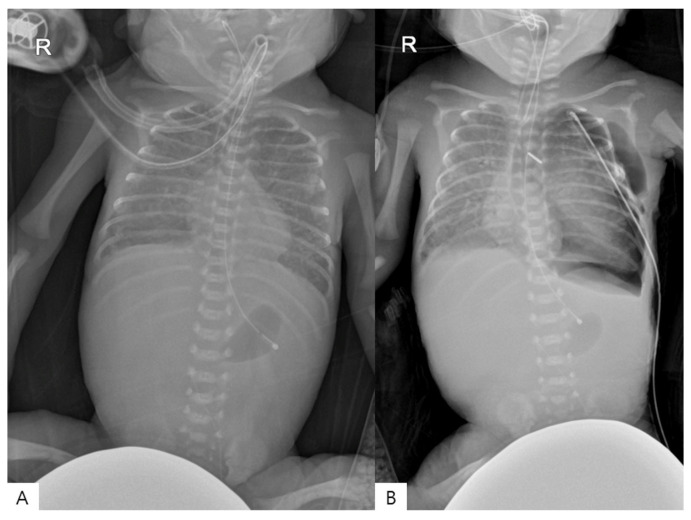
Preoperative and postoperative anteroposterior chest and abdominal radiograph. (A) Preoperative image shows diffuse bilateral lung haziness without pneumothorax. (B) Postoperative image reveals a left-side pneumothorax. R indicates the right side.
